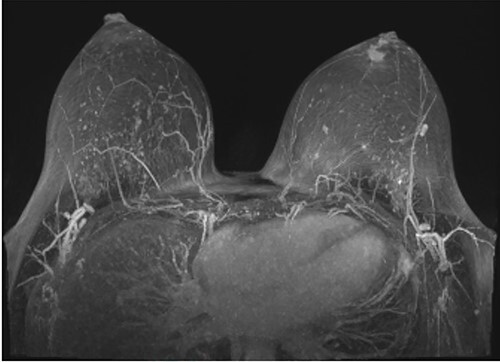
MRI of breasts showing left retro-areolar lesion.
